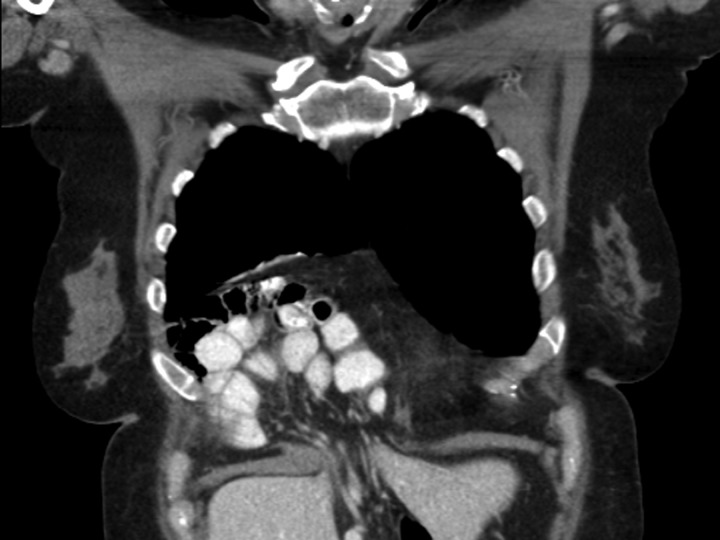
Preoperative CT scan of chest demonstrating the wide retroxyphoid defect in the diaphragm with herniated small bowel and colon into the right inferior hemithorax.
